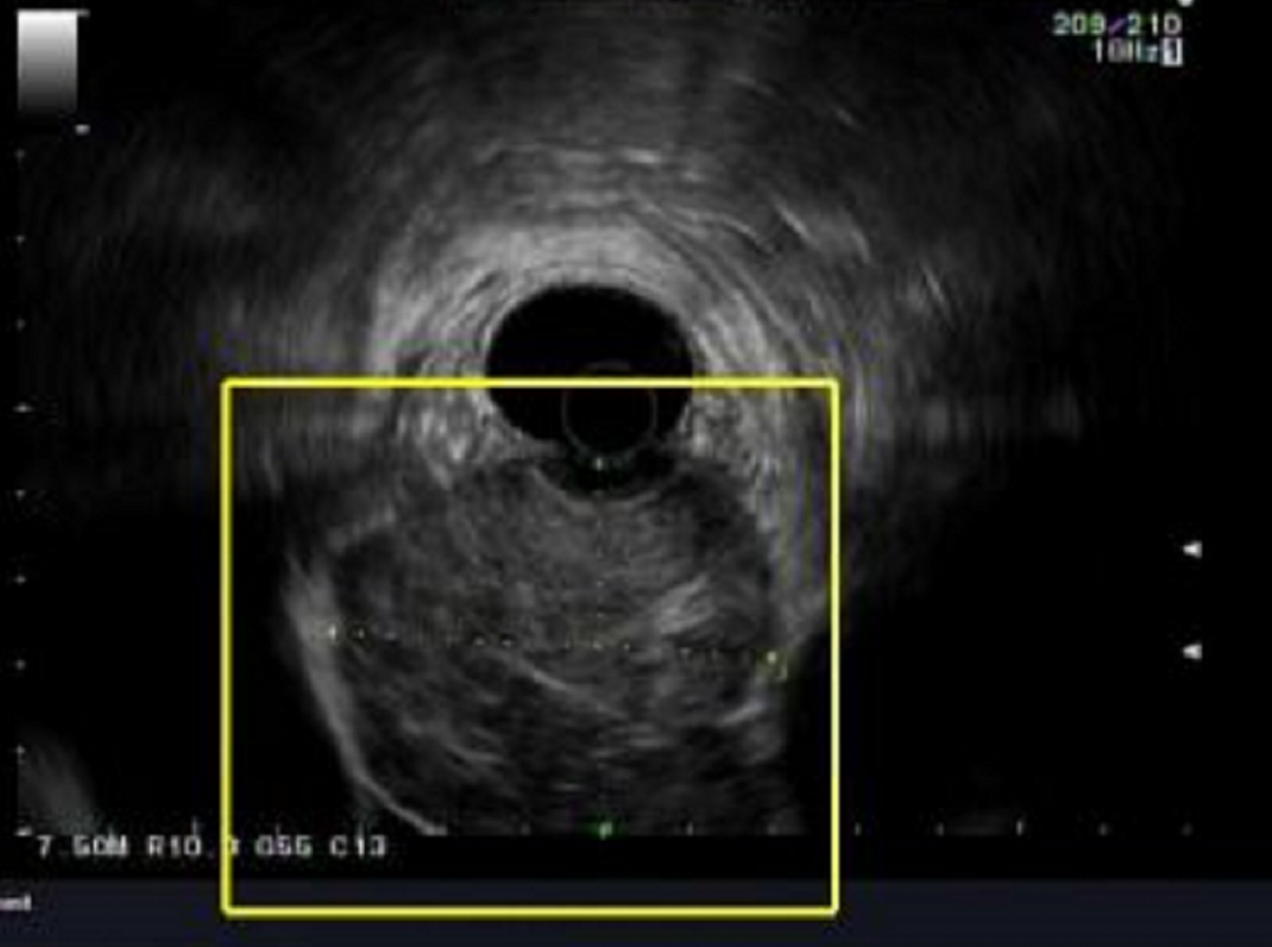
Mass measuring 68 x 55 mm abutting the gastric wall on EUS (within yellow square). EUS: Endoscopic ultrasound
